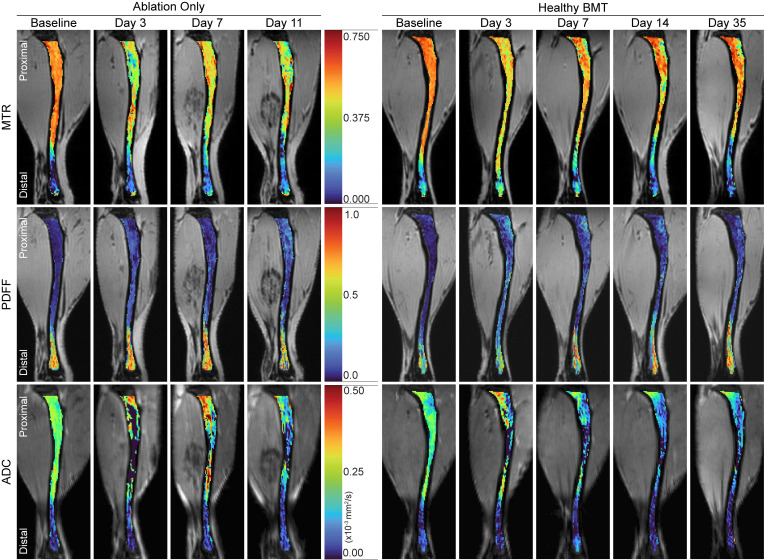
Quantitative MRI shows regional changes in BM after transplant. Representative, pseudocolored ablation-only and healthy BMT sagittal images of tibia BM showing MTR, PDFF, and ADC overlaid on corresponding, time point–matched grayscale background images (MToff; multi-gradient multi-echo mean; and low b-value, respectively). Pseudocolor scales depict range of values for each parameter. Note different ranges of values for each scale bar.
